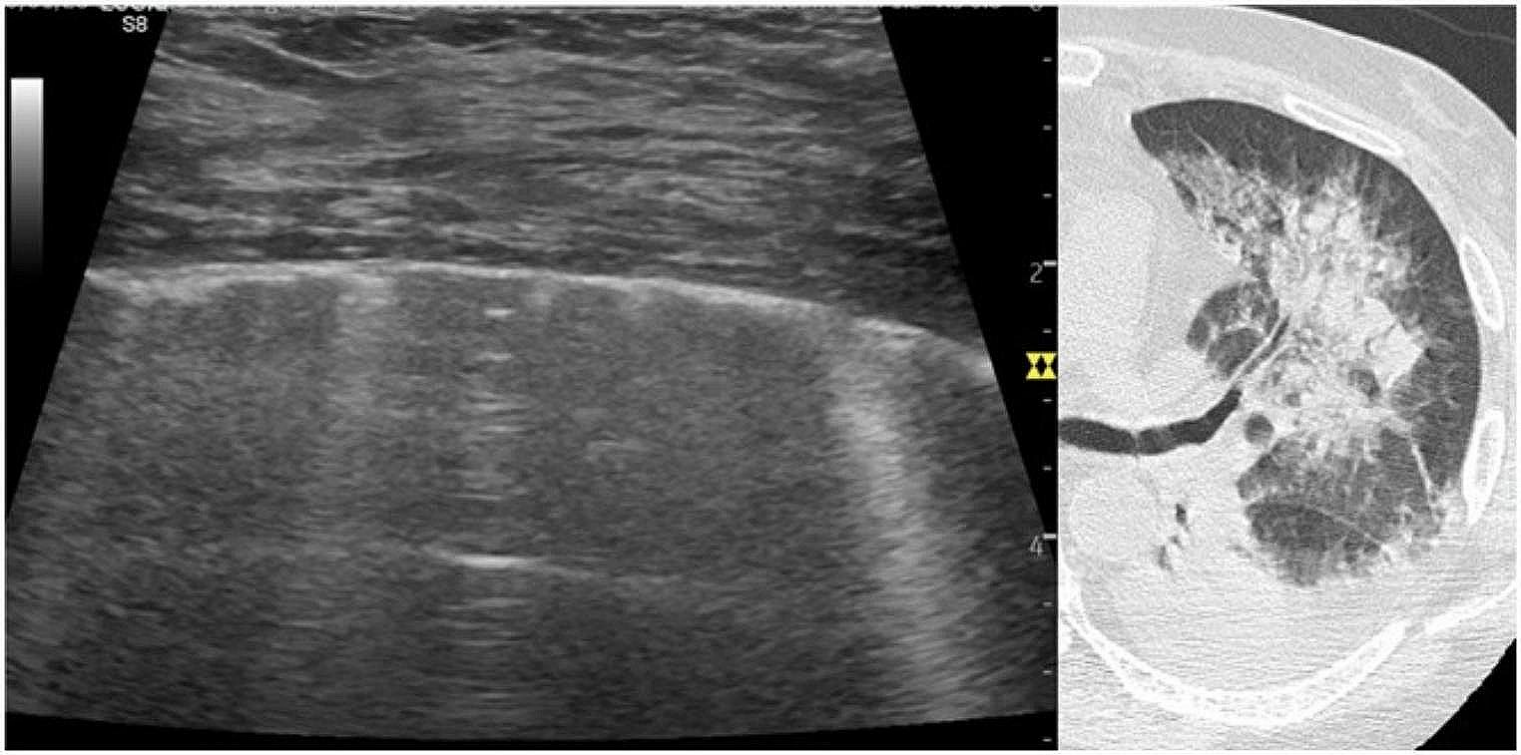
Transverse lung ultrasound approach in aspiration pneumonia with perihilar involvement. The scan was performed along the mid-axillary line (video 2 shows VLUP anterior scans from the same patient). Transverse ultrasound scan allows for visualization of a larger pleural surface without rib interruption. The pleura is only partially involved and ultrasound underestimates the damage. VLUP showed separate B-lines and small areas of confluent B-lines, without any consolidation (non-homogeneous ultrasound pattern)
